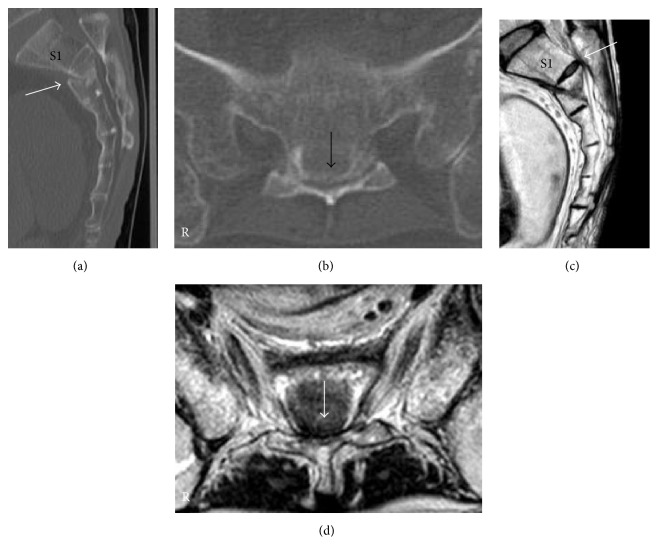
Reconstructed computed tomography (CT) and magnetic resonance image of sacrum at first hospital presentation*. (a) Sagittal reconstructed CT image. (b) Axial CT image at S2 level. (c) Sagittal plane on a T2-weighted image. (d) Axial plane on a T2-weighted image. Displaced fractures in the second sacral vertebral body (white arrow) can be observed (a). This radiological finding was consistent with a Roy-Camille classification type II fracture [5]. Sacral canal was narrowed by the protruding sacral vertebral body (black arrow) (b). This radiological finding was diagnosed as a Denis Zone III sacral fracture [15]. Sagittal and axial planes on T2-weighted images showed that sacral nerves were compressed by the vertebral body (white arrow) (c, d).
